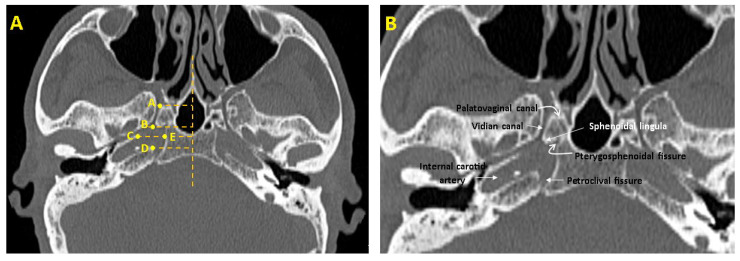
Anatomical landmarks used for morphometric measurements in the axial plane. (A) A. Anterior end of the vidian canal opening into the pterygopalatine fossa; B. Posterior end of the vidian canal opening into the foramen lacerum; C. Line drawn from the apex of the petrous part of the temporal bone to the midsagittal plane; D. Line drawn from the apex of the petrous part of the temporal bone and the petroclival fissure to the midsagittal plane; E. The point where the line extending from point C to the midsagittal plane intersects the corpus sphenoidale. (B) Anatomical structures used for measurements on CT images.
